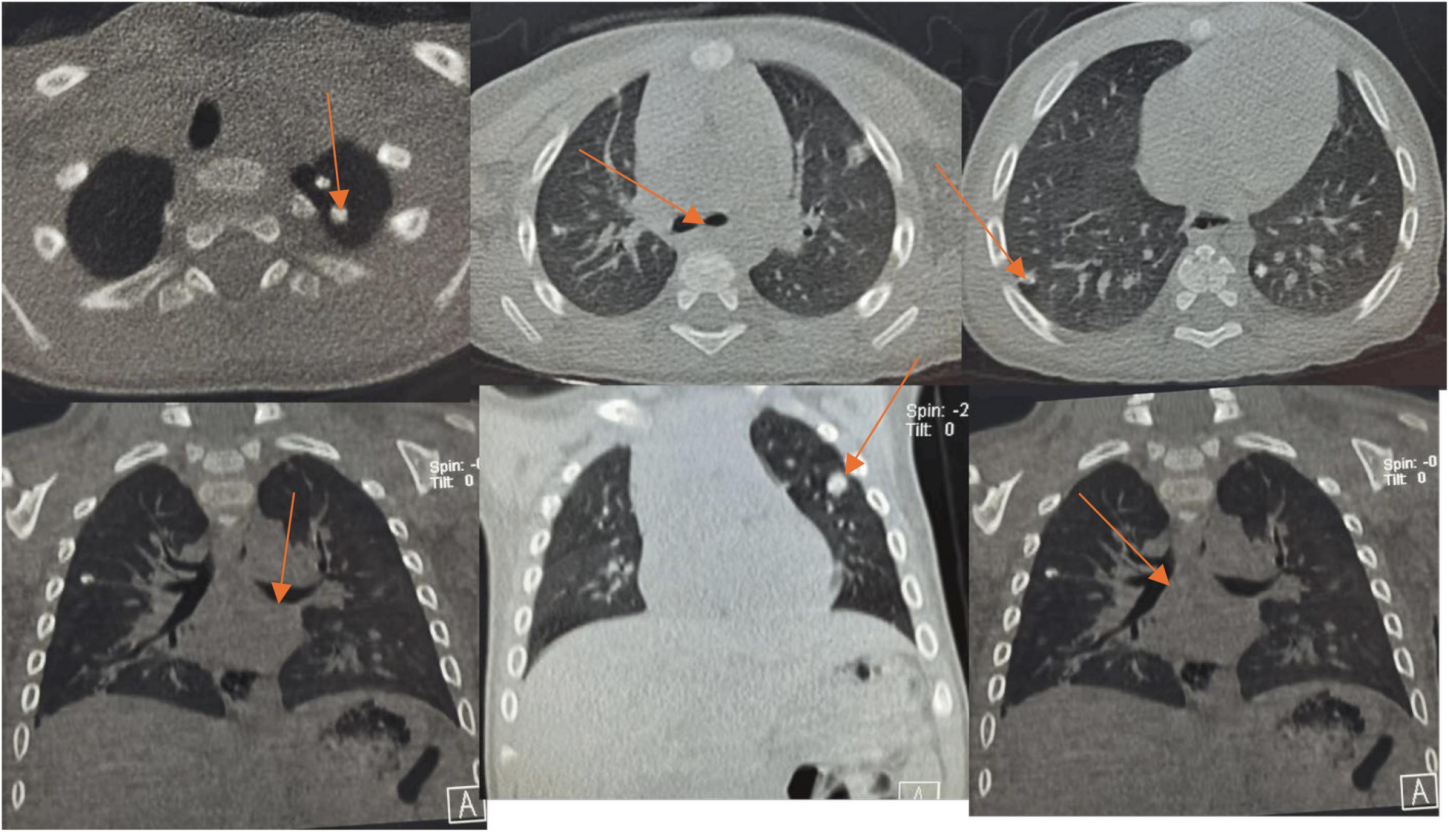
Chest CT obtained on January 17, 2025 showed complete lung re-expansion with residual calcified nodules.
